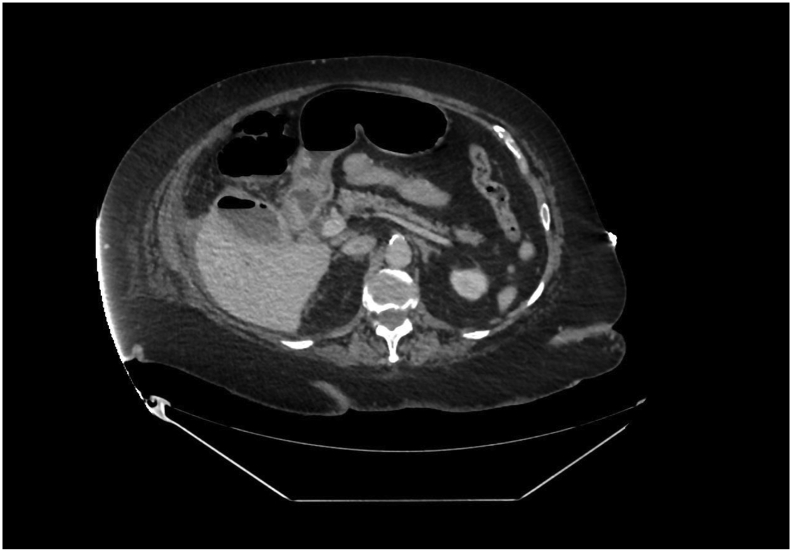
CT abdomen pelvis on admission. Axial slice of CT abdomen pelvis on admission demonstrating air fluid level within gallbladder.
